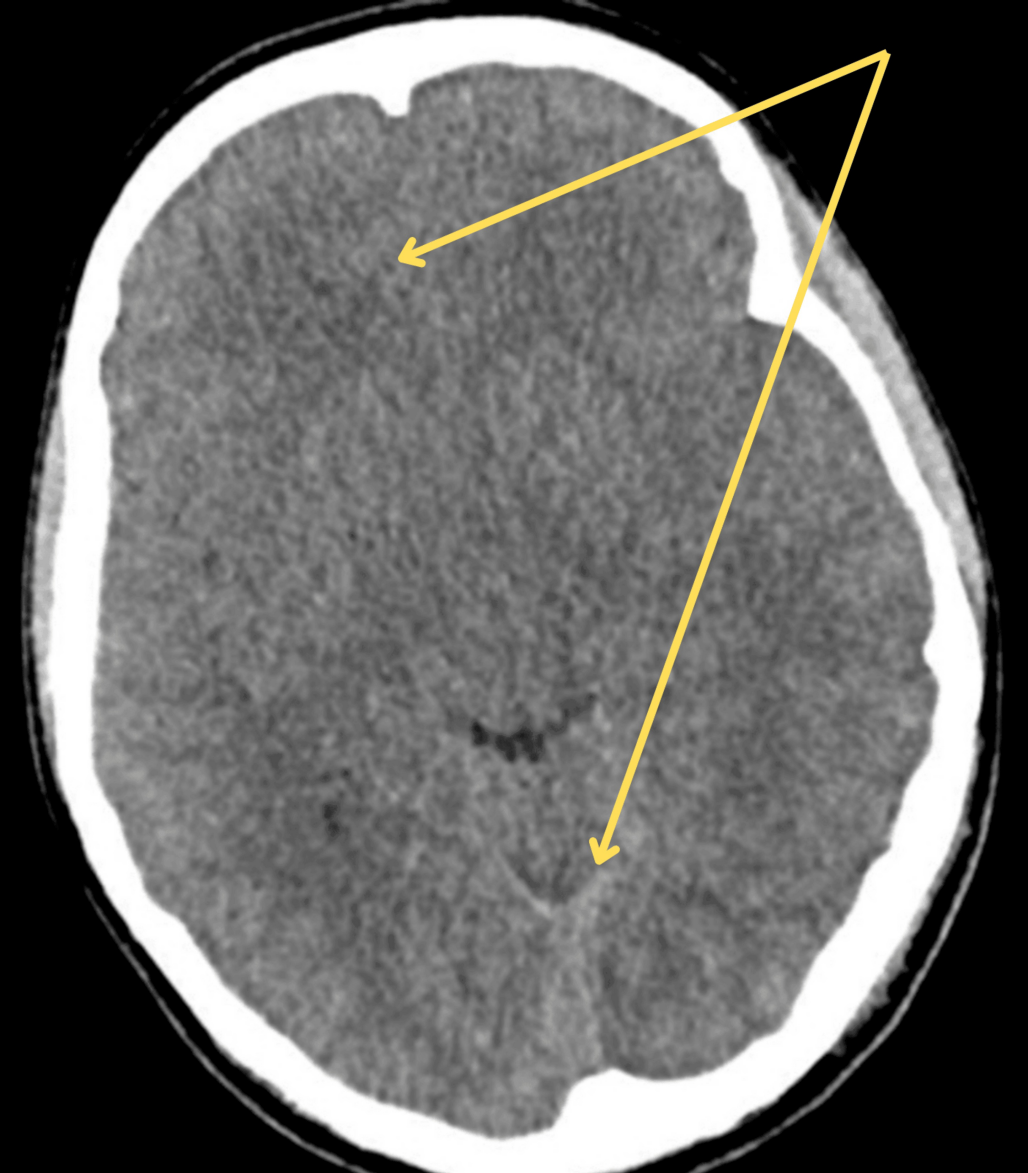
Non-contrast computed tomography (CT) of the brain showing diffuse cerebral edema with effacement of sulci and compressed ventricles, consistent with early signs of raised intracranial pressure There are marked effacement of cortical sulci, compressed lateral ventricles, and reduced gray‑white matter differentiation, findings consistent with elevated intracranial pressure.
